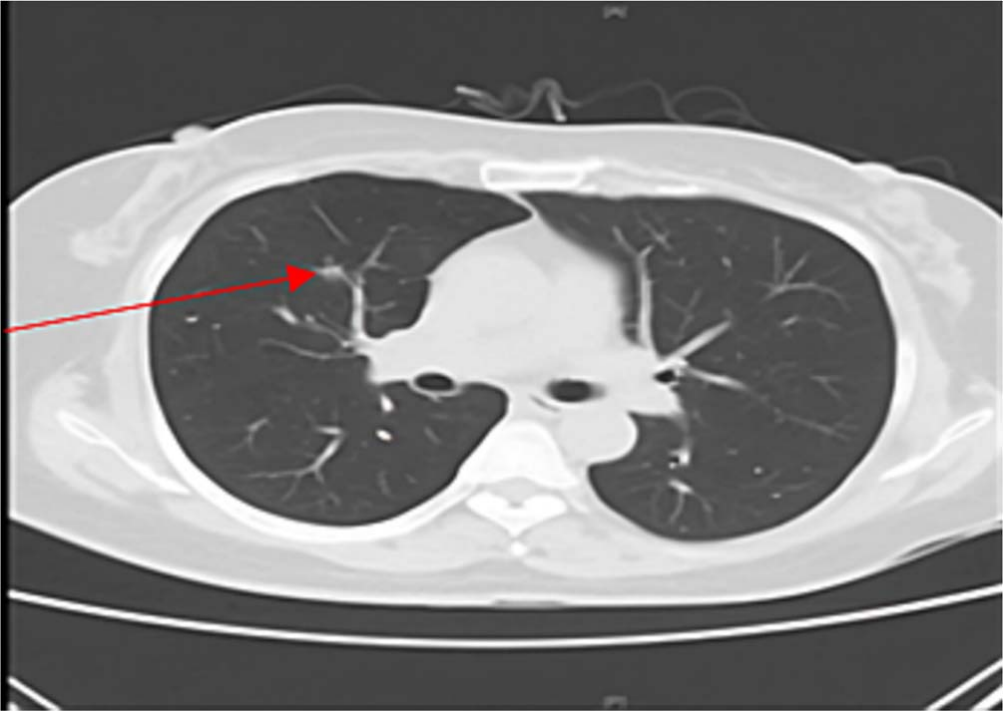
CT scan of the chest showing right middle lobe nodule.
